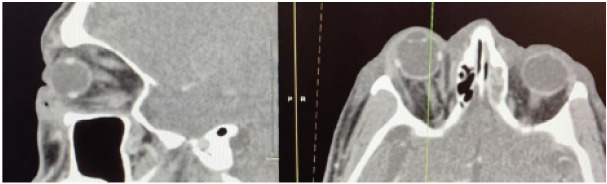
Computed tomography of the orbits with the axial view on the right and sagittal view on the left. The vertical yellow line delineates the sagittal plane shown on the left. Proptosis of the right eye can be seen on the axial view without evidence of retrobulbar hematoma or active hemorrhage.
